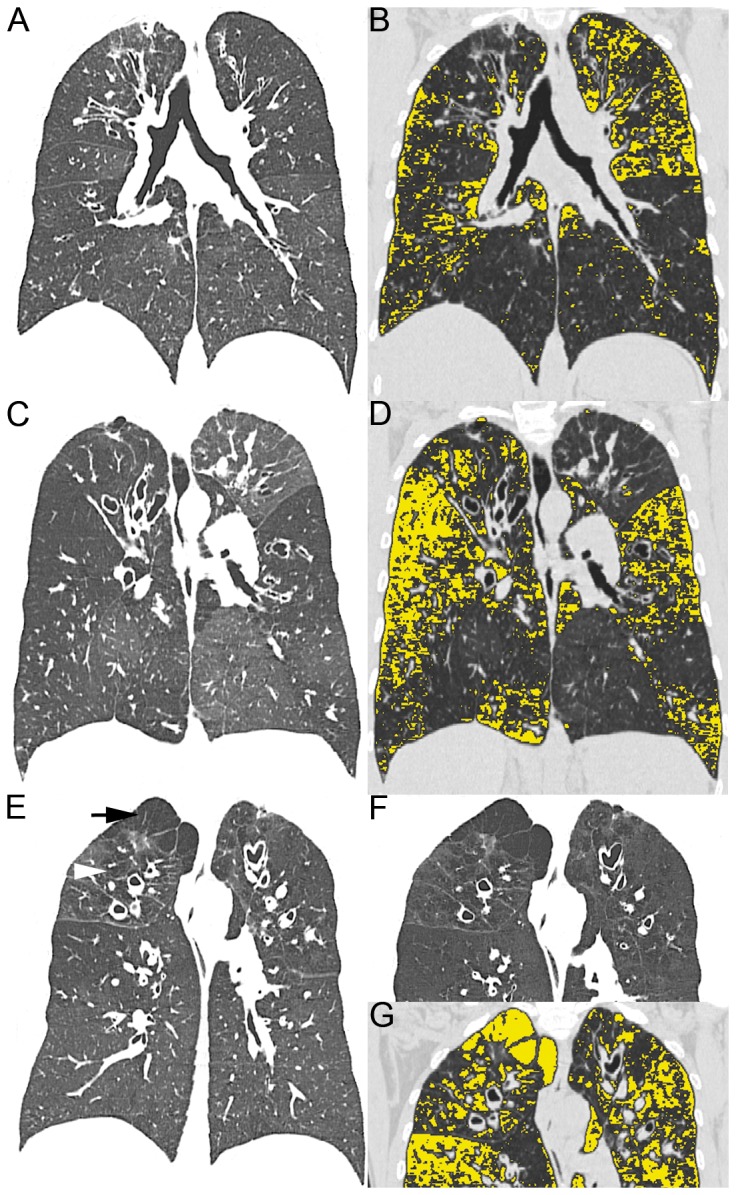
Visualization of emphysema distribution in cystic fibrosis (CF) patients by chest MDCT density masks. (A–G) Representative examples of morphologic images from non-enhanced multidetector computed tomography (MDCT) of the chest (left panels A, C, E) are complemented by density maps generated by dedicated software highlighting low attenuation areas below -950 Hounsfield units (HU) in yellow (right panels B, D, G). (A,B) MDCT image of a 36 year-old female CF patient with FEV1% = 48% showing bronchiectasis (A) as well as hypodense areas corresponding to emphysema (EI = 13.2%) mainly along subpleural and bronchovascular structures (B). (C,D) 38 year-old male CF patient with FEV1% = 29% with the lung parenchyma of the upper segments of the inferior lobes showing an overall hypodense texture and constricted vasculature (C). The density map shows extensive emphysema (EI = 24.0%) of both lungs with an emphasis on the lower lobes (D). (E–G) 46 year-old male cystic fibrosis patient (FEV1% = 55%) with marked bullous paraseptal emphysema of the right lung apex (black arrow) and centrilobular emphysema predominantly of both upper lobes (white arrowhead) (E). Note that these bullae do not possess walls differentiating them from cysts or sacculations (compare Figure S1). The minimum intensity projection (MinIP, 5 mm slice thickness) emphasizes emphysema visualization by accentuating low attenuation areas (F) with an overall EI of 18.0% (G).
